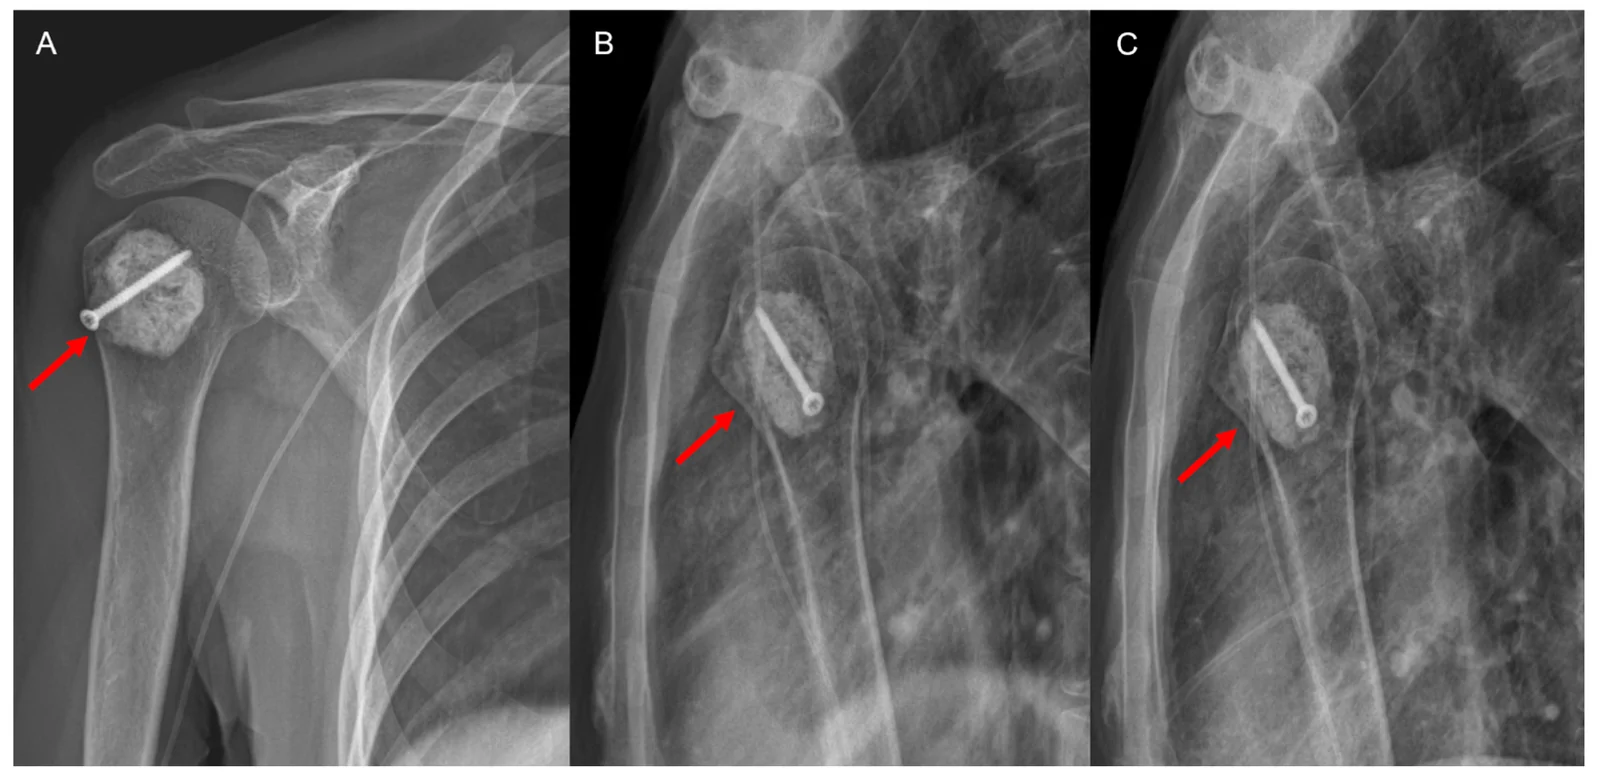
Shoulder radiographs of a 57-year-old male patient with a humeral head tumor. (A) The anteroposterior shoulder radiograph showed the postoperative region of the proximal humerus (red arrow). Compared with the transthoracic lateral radiograph acquired by the free-breathing method (B), the corresponding lateral radiograph acquired using the deep-inspiration breath-hold technique (C) showed increased lung aeration and reduced soft-tissue superimposition, with clearer delineation of the proximal humeral cortex and implanted screw (red arrow).
